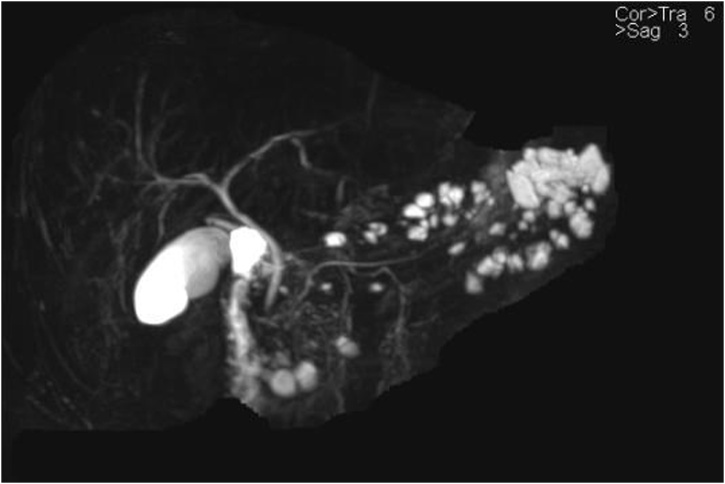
MRI of the pancreas shows diffuse multifocal cystic lesions confined to body-tail and uncinate prosses of the pancreas with no communication to PD.
